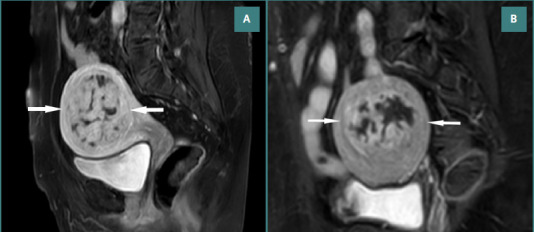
Pre-embolization enhancement of uterine fibroids on pelvic MRI. A, Sagittal fat-suppressed contrast-enhanced T1-weighted pelvic MRI demonstrating approximately 90% enhancement of a uterine fibroid. B, Sagittal fat-suppressed contrast-enhanced T1-weighted pelvic MRI showing nearly 80% enhancement of a uterine fibroid.
